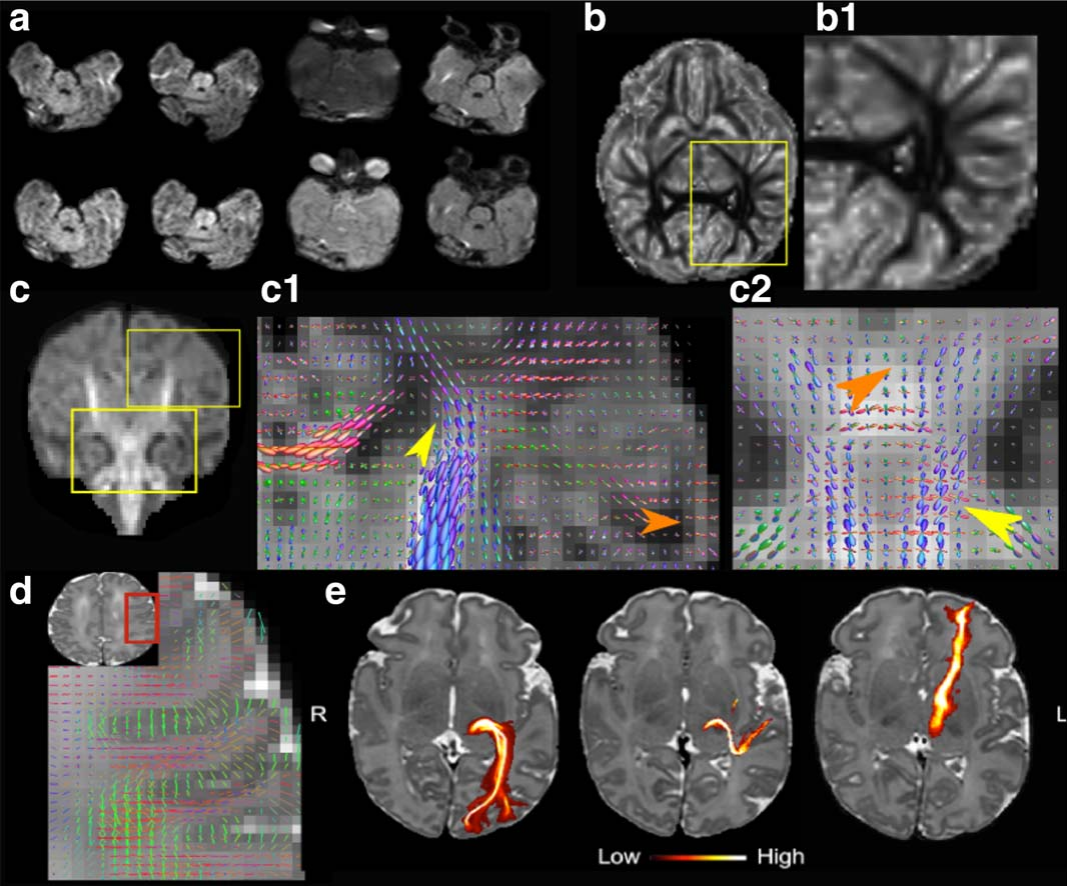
Results from the dHCP protocol showing native and distortion corrected images in four exemplary slices (a). Analysis results after processing with different algorithms: (b) Orientation dispersion index maps obtained using NODDI with a zoom to the white matter (b1) (c) Average DWI map obtained with constrained spherical deconvolution (MRTrix3) with two detailed views (c1–c2) and (d) using BEDPOSTX. (e) Probabilistic tractography results for three subcortical projections.
